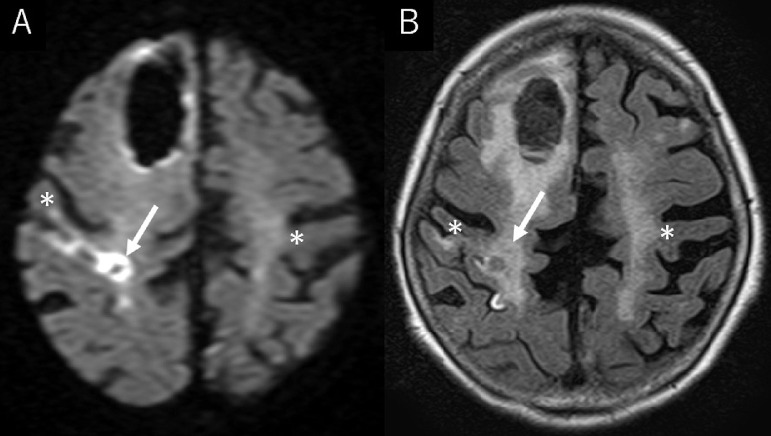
Axial diffusion-weighted magnetic resonance images showing a hyperacute cerebral infarct in the right precentral gyrus, identified as the hyperintense area (A*,* arrow), which is indistinct on the fluid-attenuated inversion recovery sequence (B*,* arrow). *Asterisk:* precentral gyrus.
